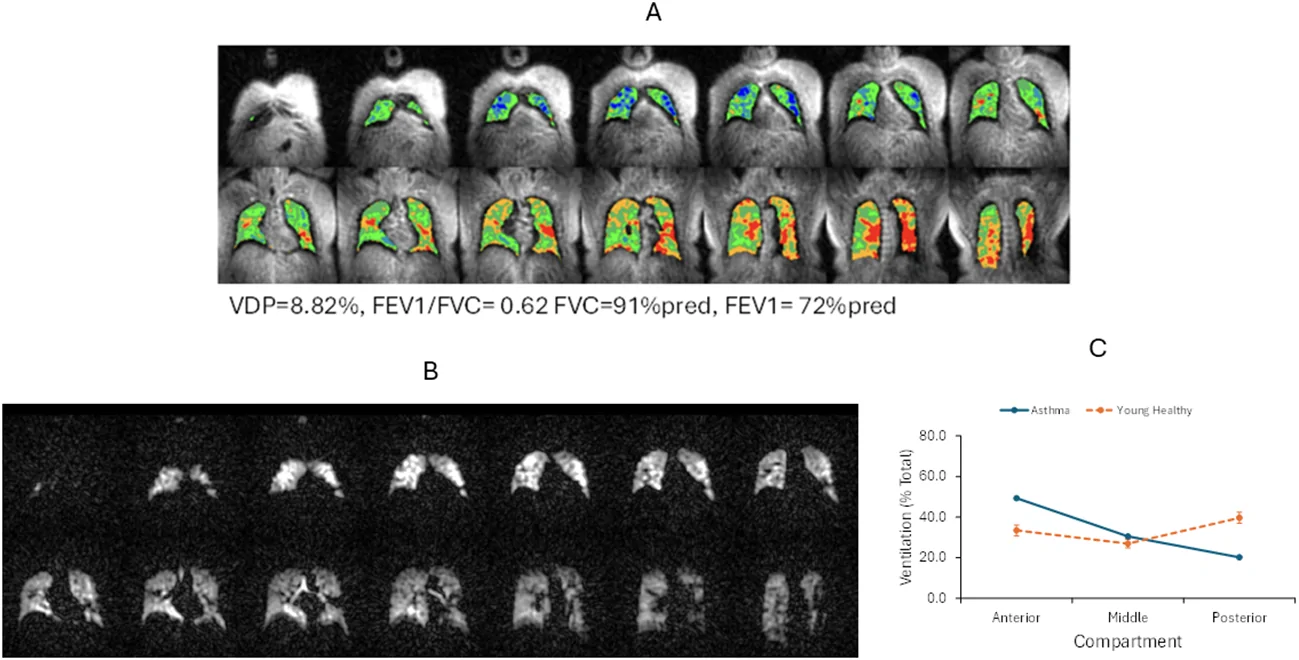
(A) MRI images processed by the N4ITK method of a 55 years old female patient with asthma who had both abnormal apical-basal and anterior-posterior ventilation. Distributions. Their anterior-posterior distributions are shown here. Red: regions with ventilation defect (VDP: >2 SD below the mean of a reference young health population. Orange: low ventilation regions (1–2 SD below the mean). Green: normal ventilation regions (mean ±1 SD). Blue: high ventilation regions (>1 SD above the mean). (B) Gray scale MRI images of the same subject processed by the RF method. (C) The three-compartment model of ventilation distribution in the anterior-posterior direction. Blue line: the asthma patient. Dotted orange line: younger healthy control.
